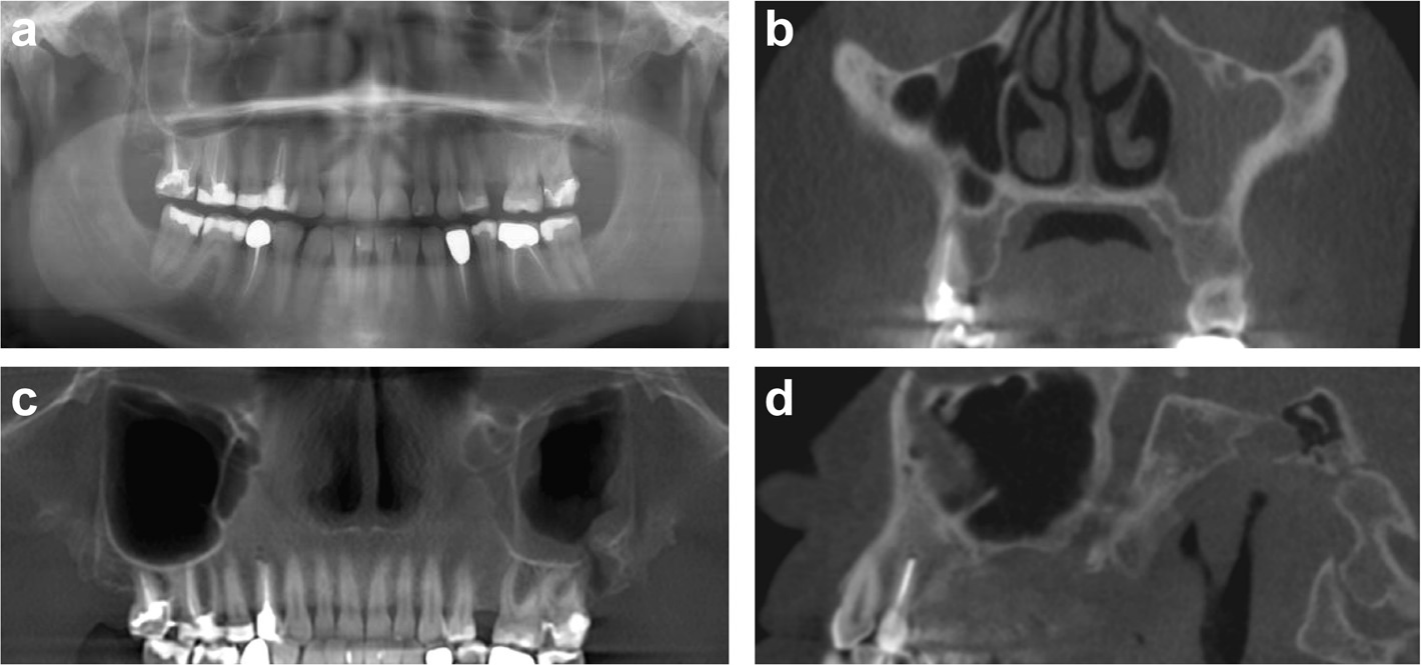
Radiographic findings of patient 19: oro-antral communication, basal opacity, basal septa; a panoramic radiography (Soredex, Cranex); b–d CBCT images (KaVo 3D eXam): coronal plane (b); panoramic reconstruction view (c); CBCT sagittal plane, right sinus (d)
